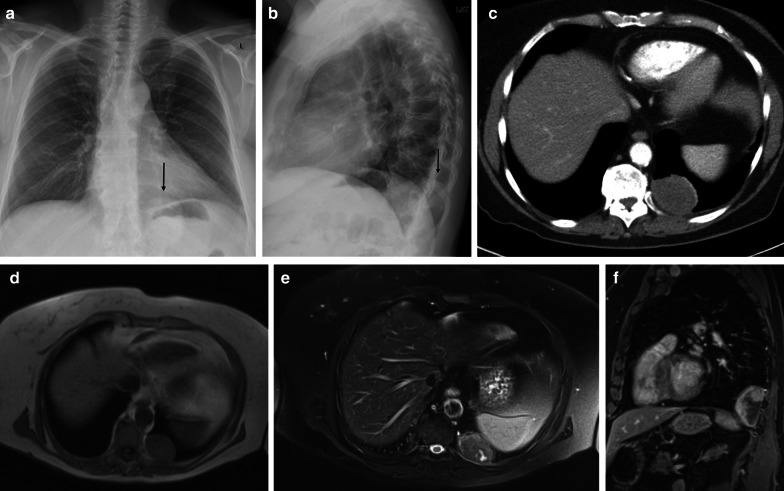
Diagnosis: solitary fibrous tumor. Technique: standard chest radiography, contrast-enhanced chest CT and MRI. Description: A 68-year-old woman presented with progressive complaints of dyspnea, cough, and limited weight loss. Postero-anterior and lateral radiographs (a, b) show a well-delineated mass (arrow) located posteriorly in the left lower lobe. Axial contrast-enhanced CT in the mediastinal window setting (c) shows a well-delineated homogeneous pleural-based mass. There is no invasion of the surrounding structures. On the axial T1-WI MR image (d), the lesion has a homogeneous isointense signal intensity to muscle. On the axial T2 blade FS MR image (e), the lesion has a low signal intensity with peripheral enhancement on the sagittal T2W FS MR image after i.v. contrast administration (f)
